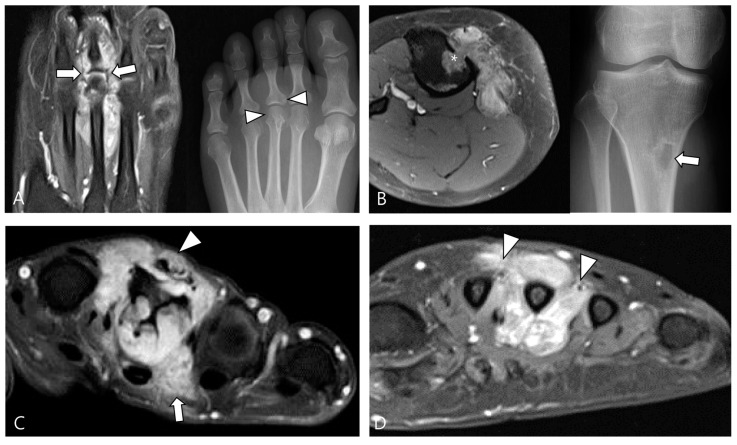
Involvement of adjacent structures of D-TSGCT on contrast-enhanced fat-suppressed T1WIs. (A) Articular involvement (arrows) of the metatarsophalangeal joint of the foot, also correlated on a plain radiograph (arrowheads) presenting as a periarticular bony change. (B) Bone erosion (asterisk) of the tibia, also correlated on a plain radiograph (arrow). (C) Tendon (arrowhead) and muscular involvement (arrow) with infiltration into the extensor tendon fibers and interosseous muscle of the foot. (D) Neurovascular bundle involvement (arrowheads) with more than 180° encasement of the neurovascular bundle of the foot.
